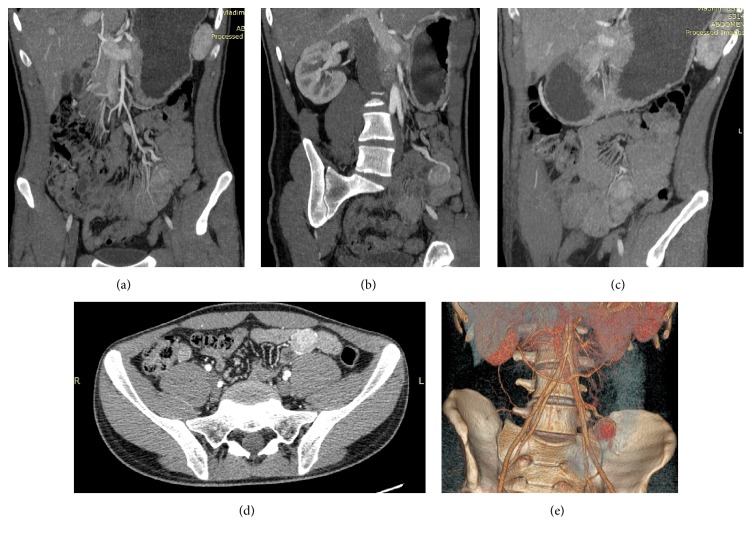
Contrast-enhanced CT showing highly vascular small bowel neoplasm: (a) coronal section, (b) and (c) oblique sections, (d) axial section, and (e) 3D reconstruction.
